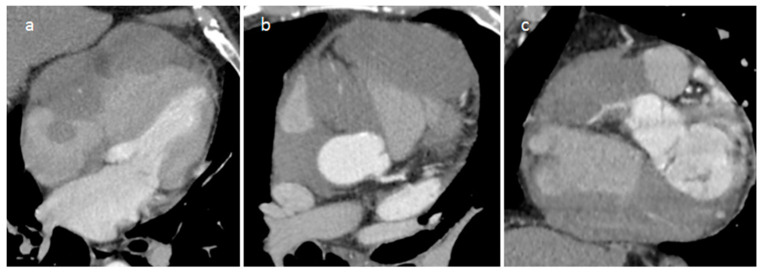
A 63-year-old male patient with right heart large B-cell lymphoma on four-chamber (a), axial (b) and short-axis CT (c) views.
